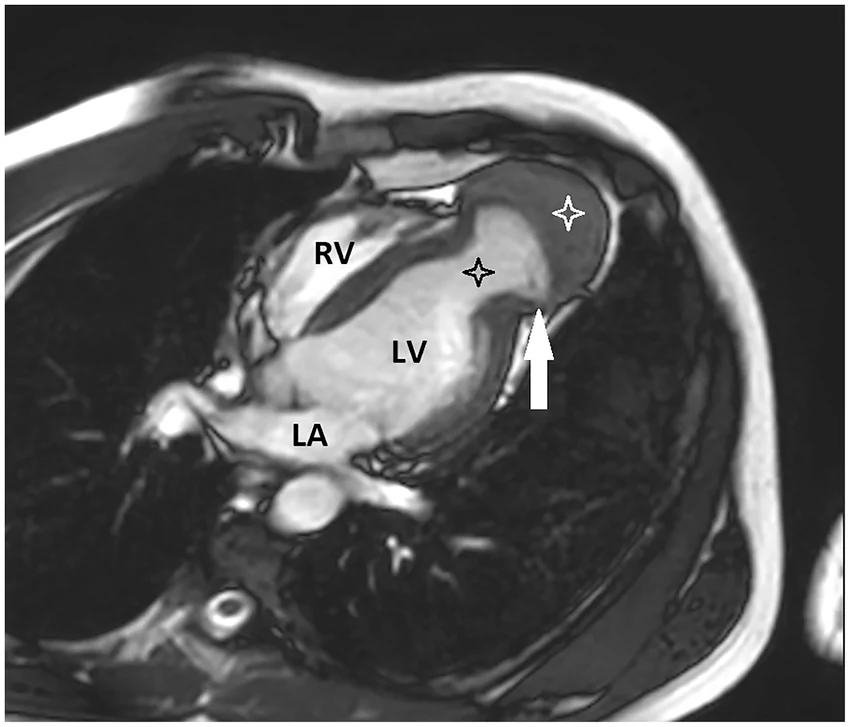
Patient's cardiac MRI image showing a left ventricular pseudoaneurysm(4-chamber cine, horizontal long-axis).
